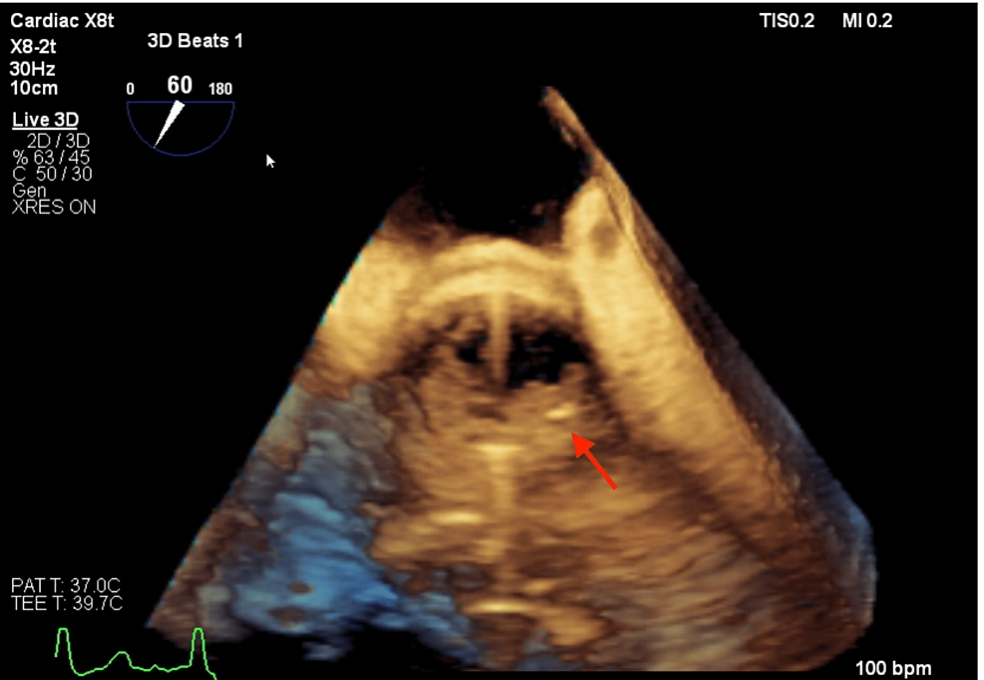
TEE showing perivalvular abscess on an aortic prosthetic valve (arrow) TEE: transesophageal echocardiography
